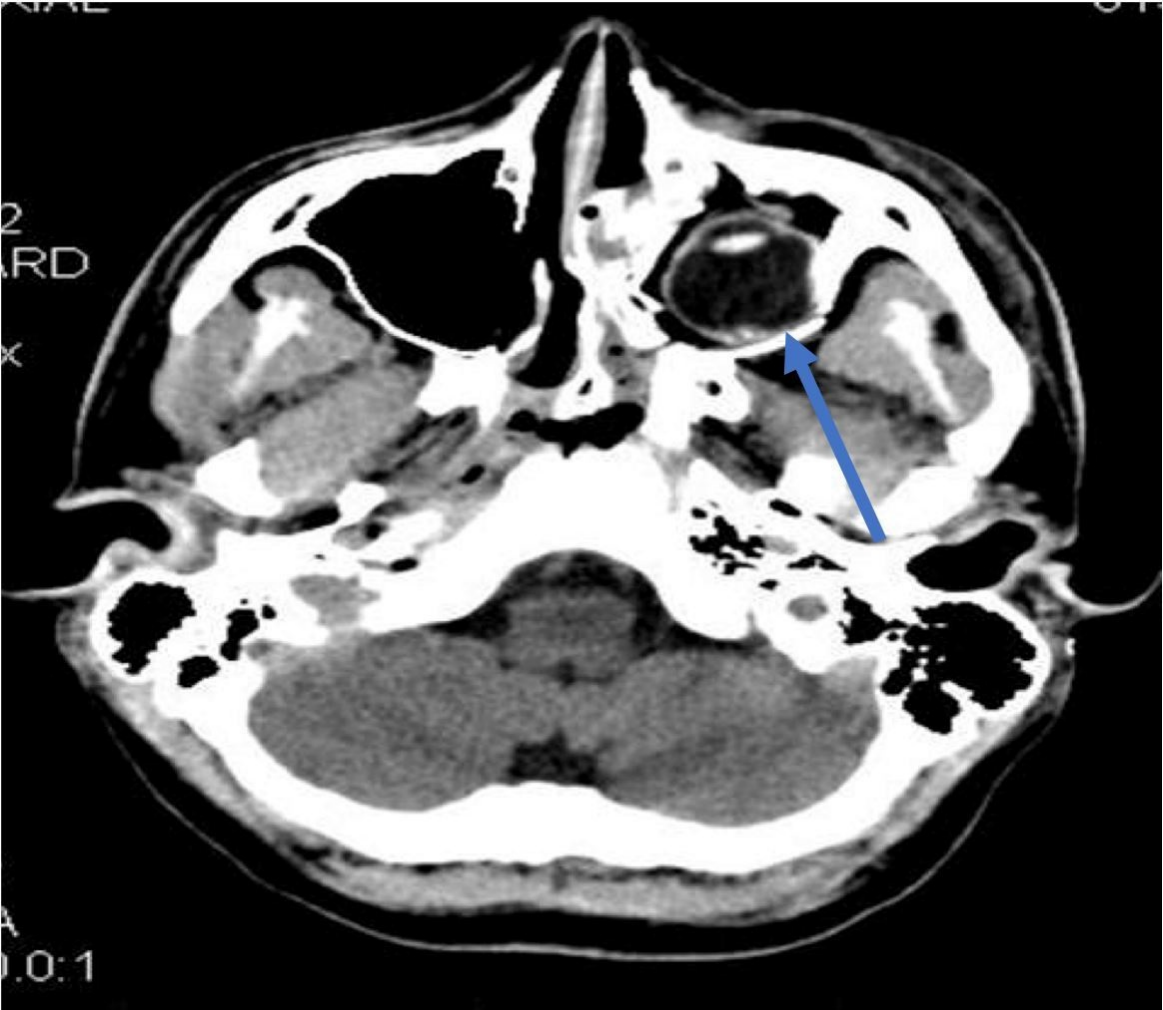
Computed tomography (CT) of head: axial view through the maxillary sinus. Blue arrow: the globe with intact lens sitting within the maxillary sinus.
